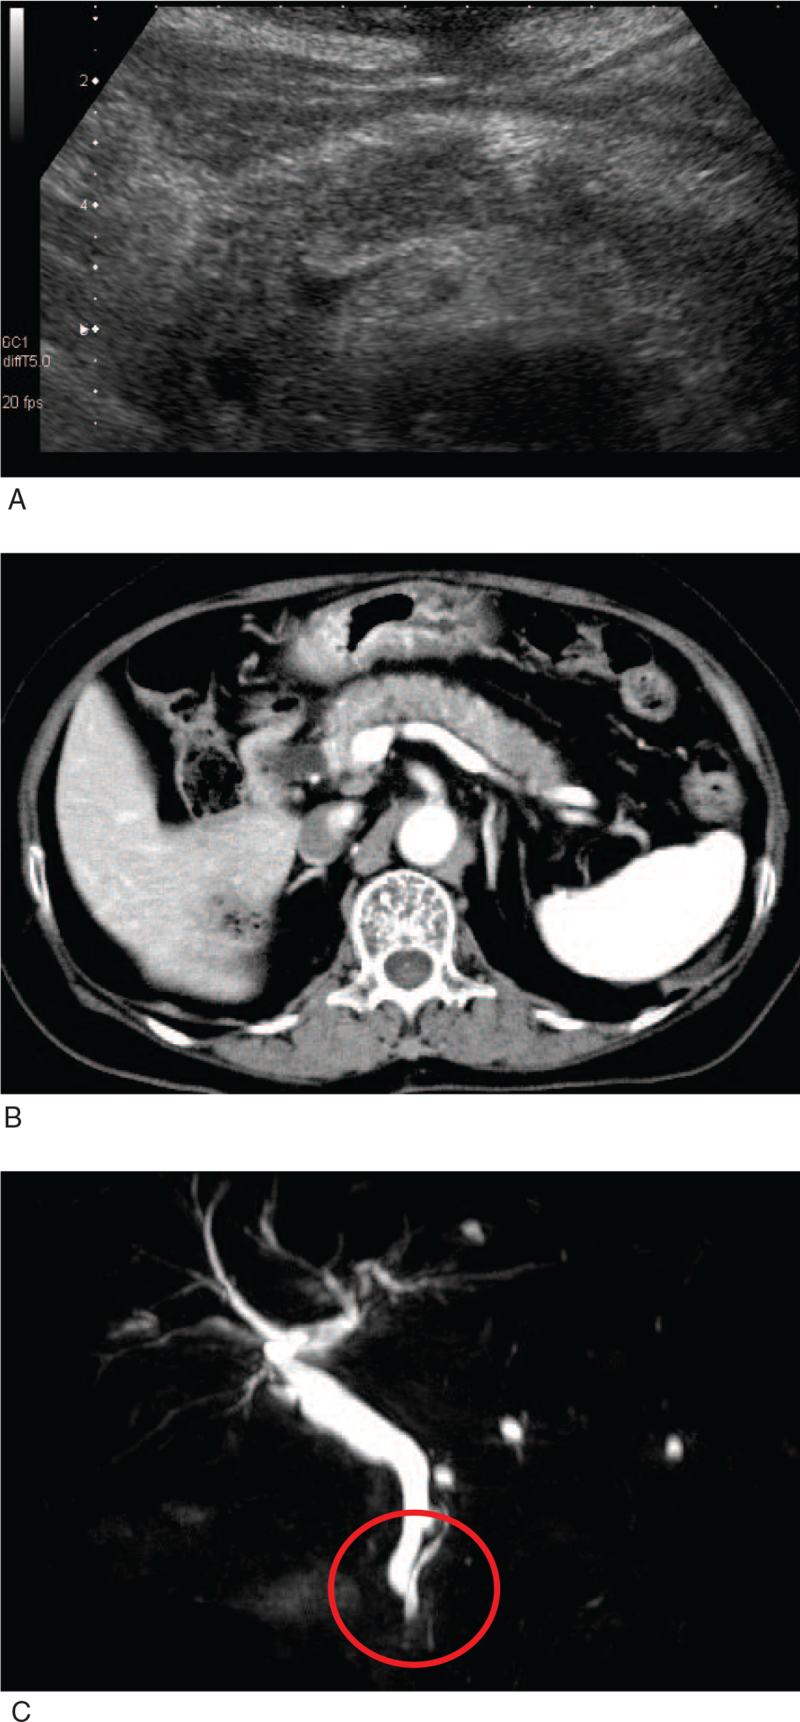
(A) Abdominal ultrasonography. Hypoechoic change of the pancreas was observed, but there was no dilation of pancreatic duct. (B) Abdominal contrast computer tomography. Diffuse swelling of the pancreas accompanied by capsule-like rim was observed. These are characteristic and important findings for the diagnosis of autoimmune pancreatitis. (C) Magnetic resonance cholangiopancreatography. Intrapancreatic common bile duct narrowing and duct type intraductal papillary mucinous neoplasms were suspected. However, there were no tumorous lesions in the pancreas and no dilation in main pancreatic duct.
